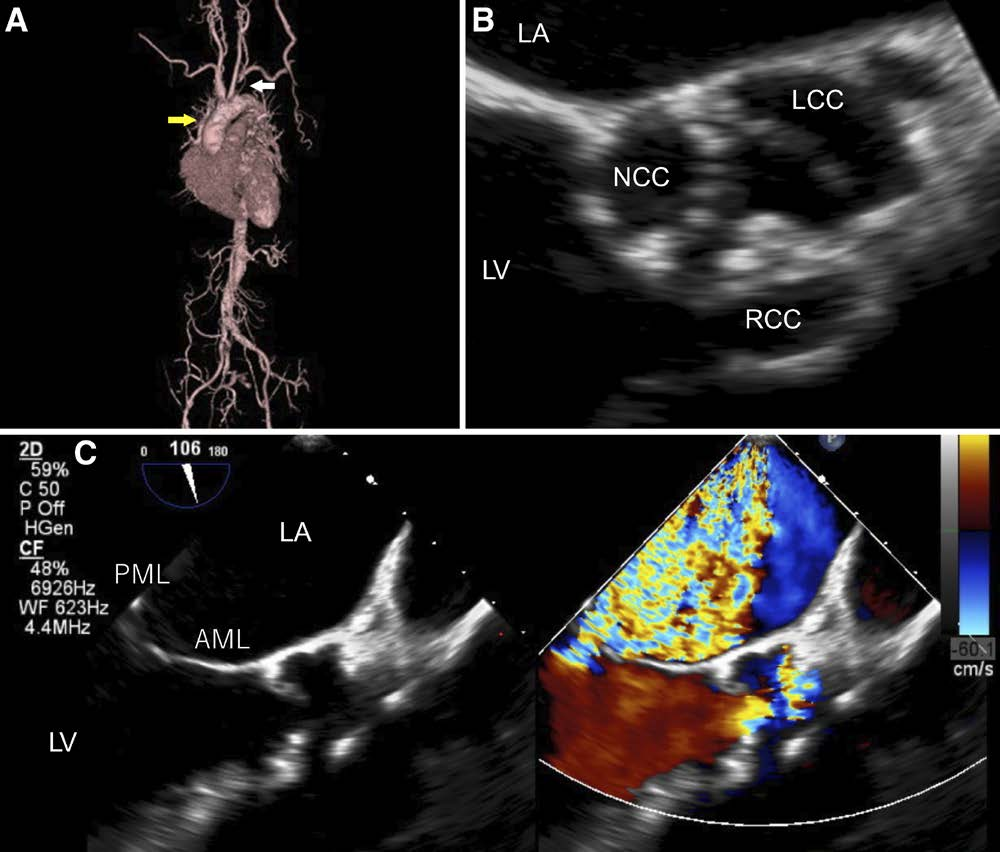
Severe aortic valve stenosis and mitral regurgitation in a patient with systemic lupus erythematosus on hemodialysis due to lupus nephritis. The patient was a 59-year-old woman with a 15-year history of SLE. She was introduced to dialysis 10 years earlier due to lupus nephritis. She also had an IVC filter implanted for deep vein thrombosis. (A) 3D-CT shows multiple atheromas around the thoracic aorta and occlusion in the left subclavian artery (white arrow). The ST junction is smaller than the annulus, and the gap is recognized below the commissure. (B and C) TEE showed severe aortic stenosis and mitral regurgitation. The opening of the RCC and NCC of the aortic valve is severely restricted due to severe calcification, and there is mitral regurgitation due to severe calcification of the posterior leaflet of the mitral valve. 3D-CT = three-dimensional computed tomography, AML = anterior mitral leaflet, IVC = inferior vena cava, LA = left atrium, LV = left ventricle, LCC = left coronary cusp, NCC = non-coronary cusp, PML = posterior mitral leaflet, RCC = right coronary cusp, SLE = systemic lupus erythematosus, TEE = transesophageal echocardiography.
